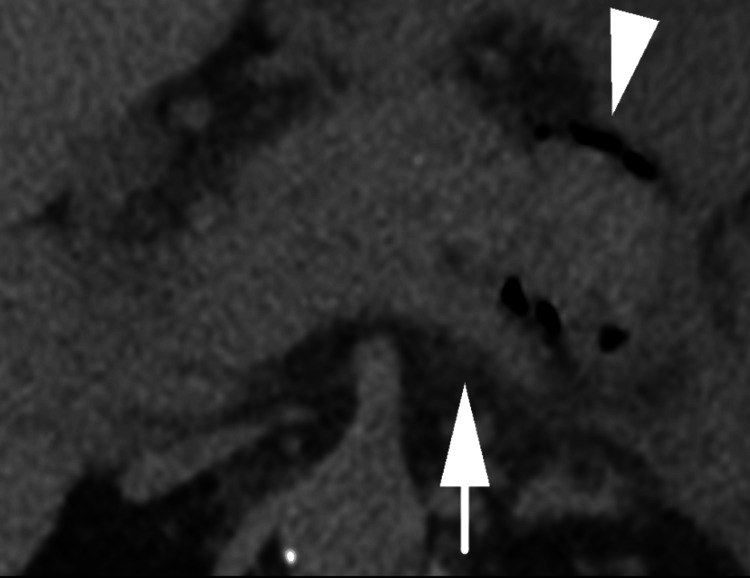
Axial non-contrast CT image of the pancreas demonstrating gas within the body of the pancreas (white arrowhead) with peripancreatic fat stranding (white arrow).
